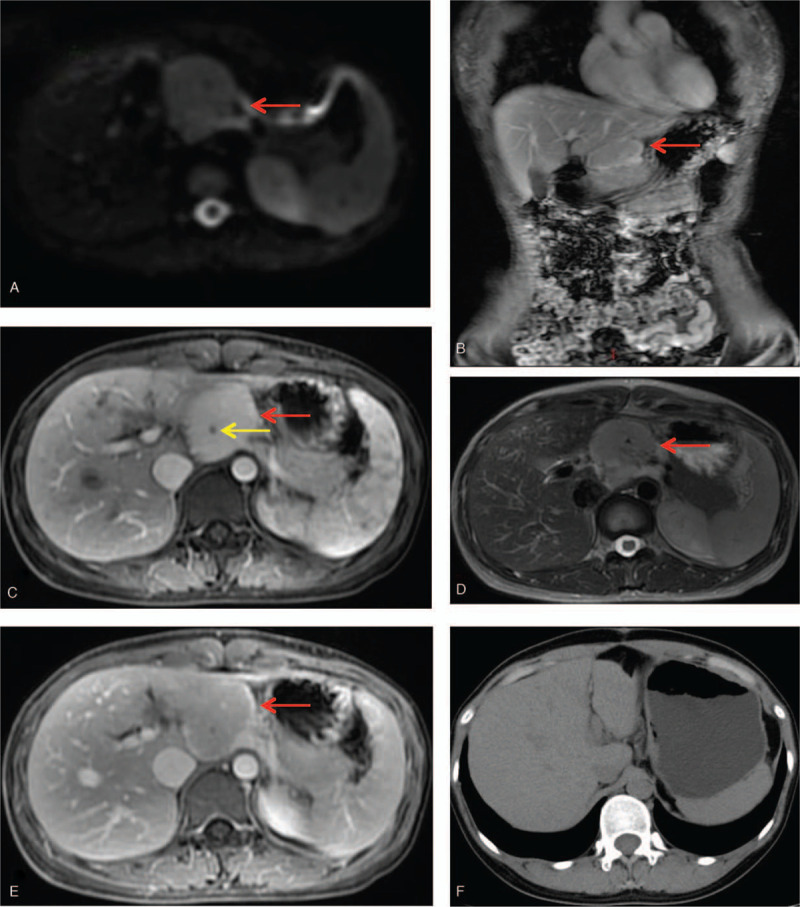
Magnetic resonance imaging of the lesion (red arrow) in Case; Microcalcifications (yellow arrow). (A) Diffusion weighted imaging; (B) coronal scan; (C) Transverse T1-weighted fat-suppression sequence (arterial phase); (D) Transverse T2-weighted fat-suppression sequence; (E) Transverse T1-weighted fat-suppression sequence (Venous phase); (F) CT images reviewed 1 yr later and the mass disappeared without recurrence.
